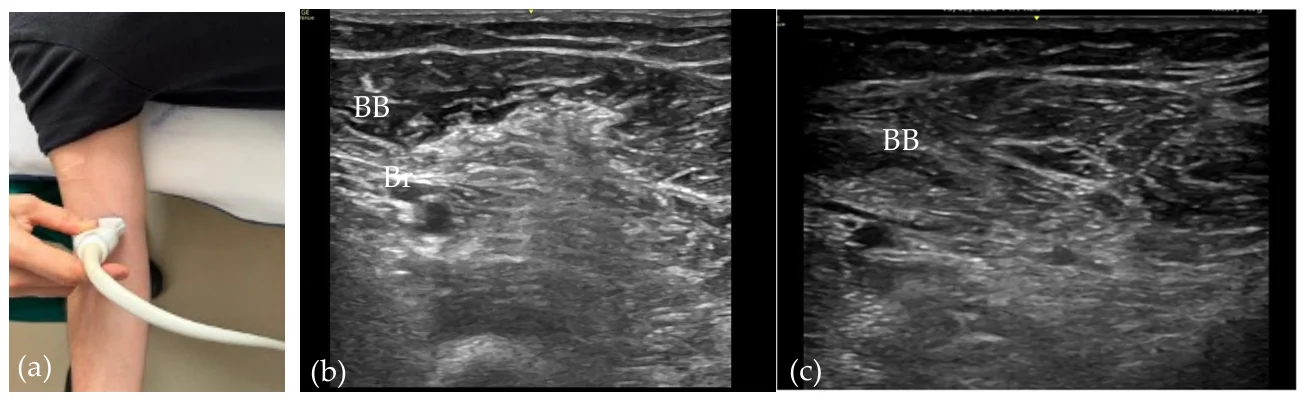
MSK US probe placement. BB, brachialis muscle and brachial artery assessed at T0 and T1. Abbreviations: BB: biceps brachii; MSK US: musculoskeletal ultrasound; T0: baseline assessment; T1:follow-up at 3 months; (a) Patient positioning and probe placement at the mid-arm level. (b) MSK US image of BB, brachialis muscle, and brachial artery at T0. Baseline ultrasound image demonstrating the superficial biceps brachii muscle and the deeper brachialis muscle adjacent to the brachial artery. Increased echogenicity and altered muscle architecture can be observed. (c) MSK US image of BB, brachialis muscle, and brachial artery at T1. Follow-up ultrasound image obtained at 3 months using the same acquisition protocol. Muscle architecture remained largely preserved, with only subtle changes in echotexture despite clinically meaningful functional improvement.
